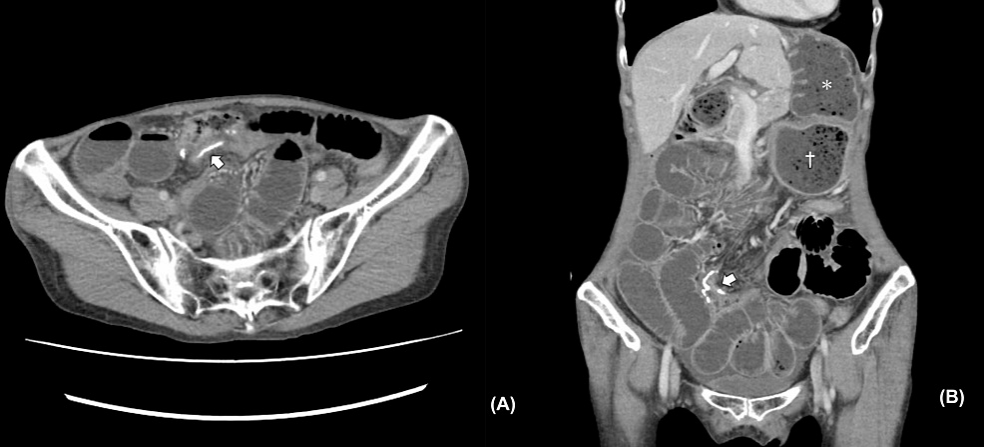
CT findings A small bowel obstruction was observed on a CT scan, but the cause is unknown. A: axial view, B: coronal view, arrow: anastomosis site of the residual ileum and the transverse colon, ＊: dilated interposed colon, †: dilated residual stomach, CT: computed tomography
